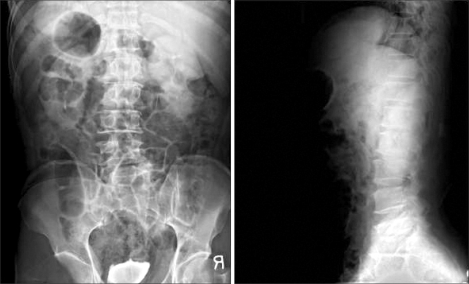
Radiograph showed axial malalignment and decreased vertebral body height of L5.
